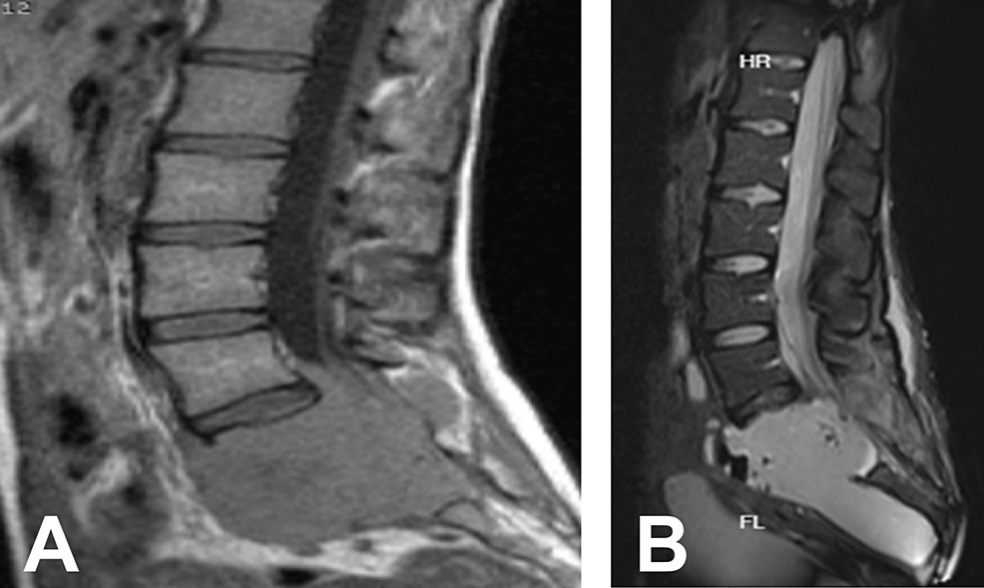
(A) Non-contrast-enhanced preoperative sagittal T1-weighted MRI; (B) postoperative MRI. Note the amount of resection and resection cavity.
